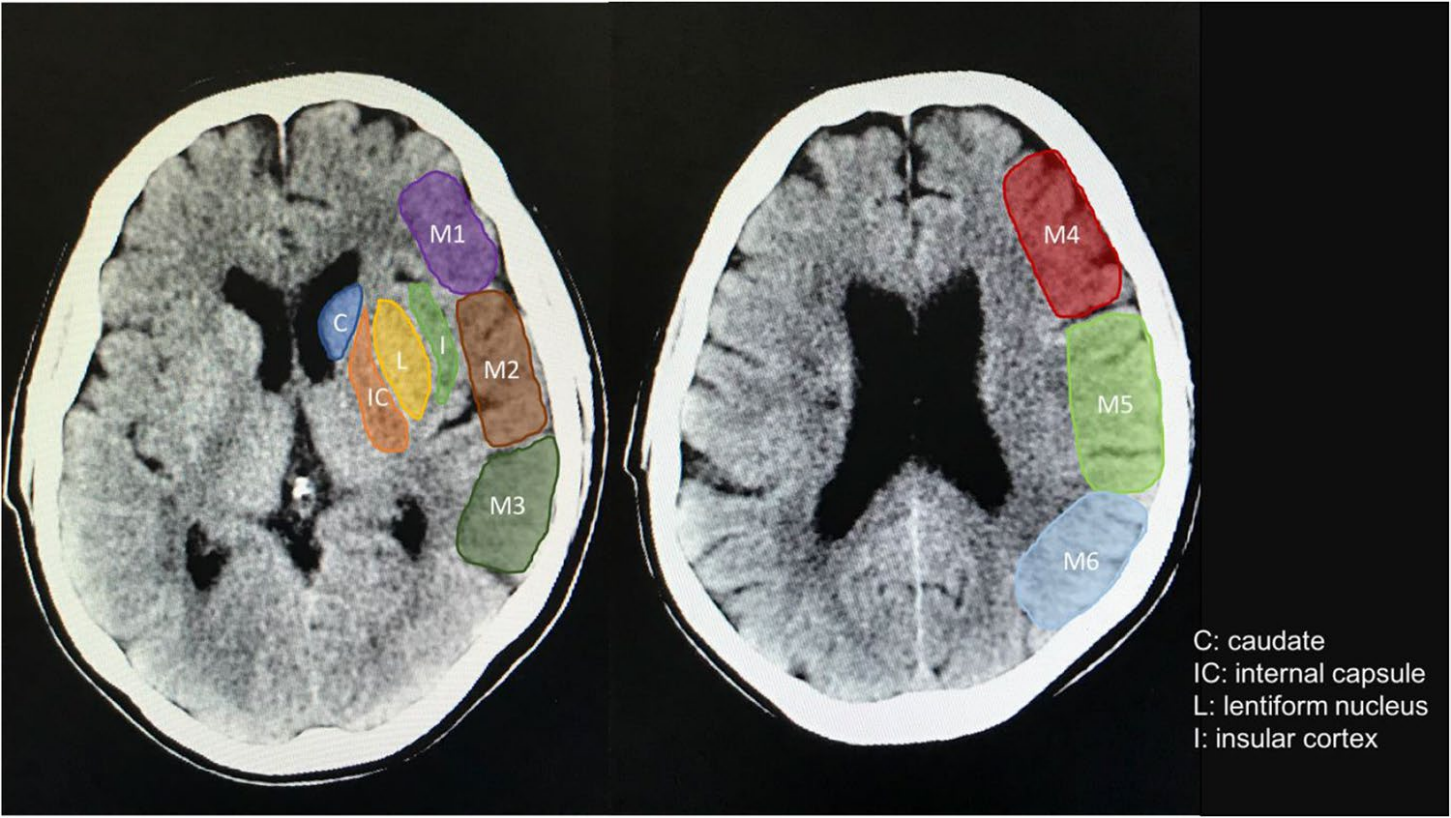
Calculating ASPECT score from non-contrast CT head images. (a) Level of the basal ganglia for visualisation of caudate, internal capsule, insula, and M1–3. (b) Level of the corona radiata for visualisation of M4–6
